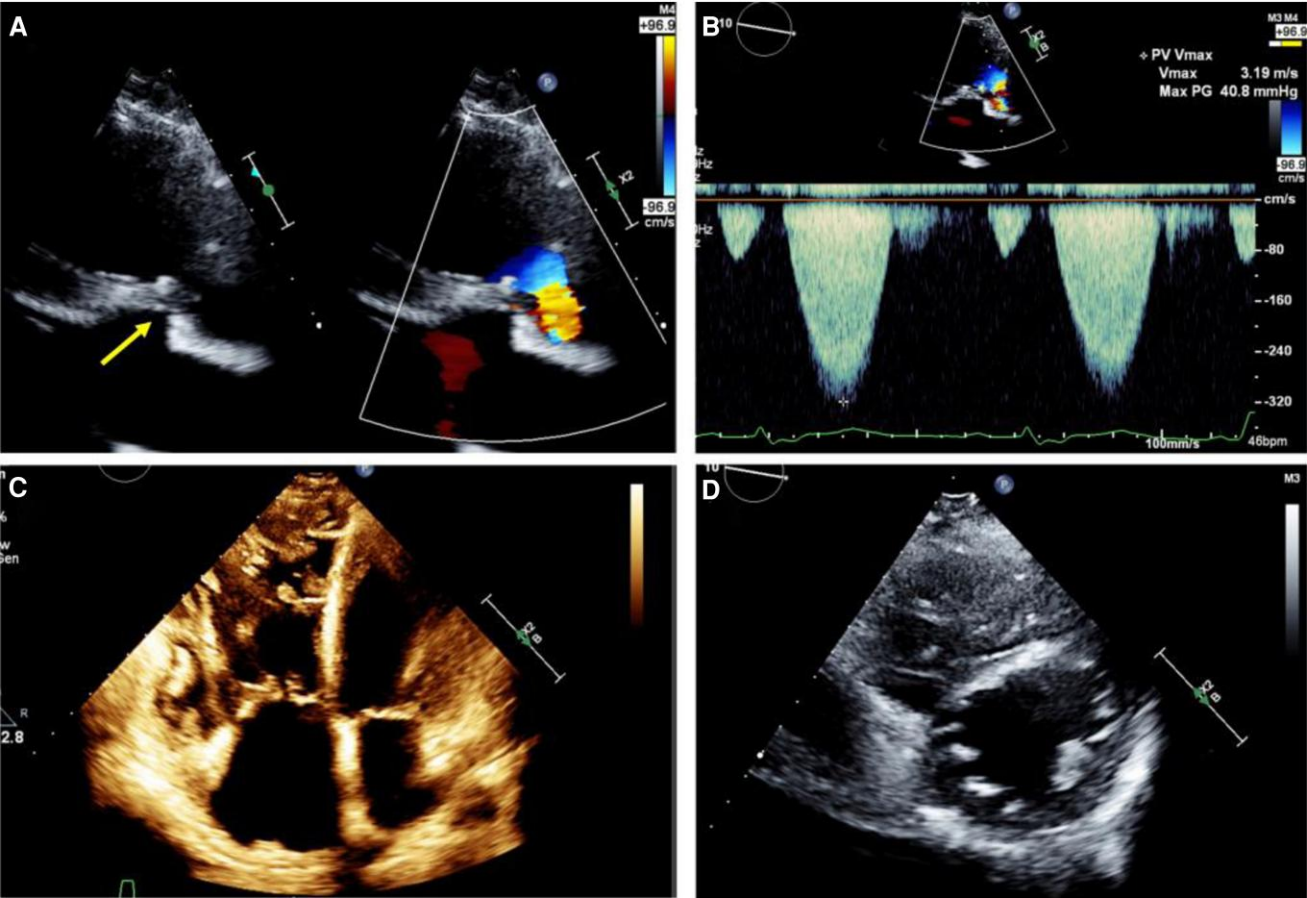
Transthoracic echocardiographic images. Two-dimensional echocardiographic image of the pulmonary artery stenosis site (arrow) (*A*) and Doppler measurement showing a peak velocity of 3.2 m/s and peak pressure gradient of 41 mmHg at the stenotic site (*B*). Apical four-chamber view and short-axis view prior to transcatheter pulmonary valve implantation demonstrating biventricular dysfunction with right ventricular pressure and volume overload (C and *D*).
